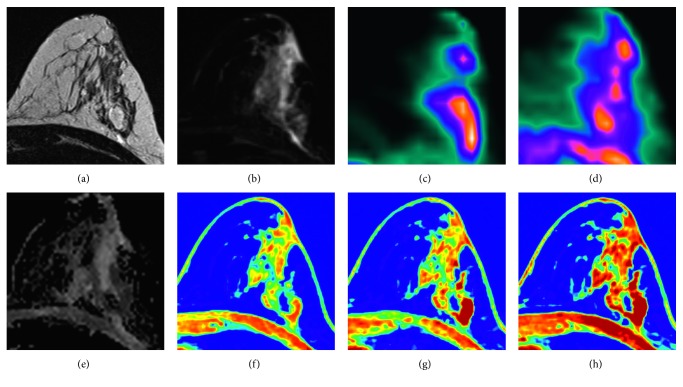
46-year-old patient with hormone receptor positive/Her2 negative invasive ductal carcinoma in the left breast. Multiparametric [^18^F]FDG/[^18^F]FMISO PET-MRI : cancer presented as a segmental heterogenous persistently enhancing nonmass enhancement. (f–h) DCE_native_, DCE_early_, and DCE_delayed_ with perifocal edema. (a) T2-weighted without fat saturation. (b) T2-weighted with fat saturation. The lesion showed moderate uptake of (c) [^18^F]FDG (SUV_max_ = 6.5) and (d) [^18^F]FMISO (SUV_max_ = 1.3) with restricted diffusion visualized on the ADC map (e).
